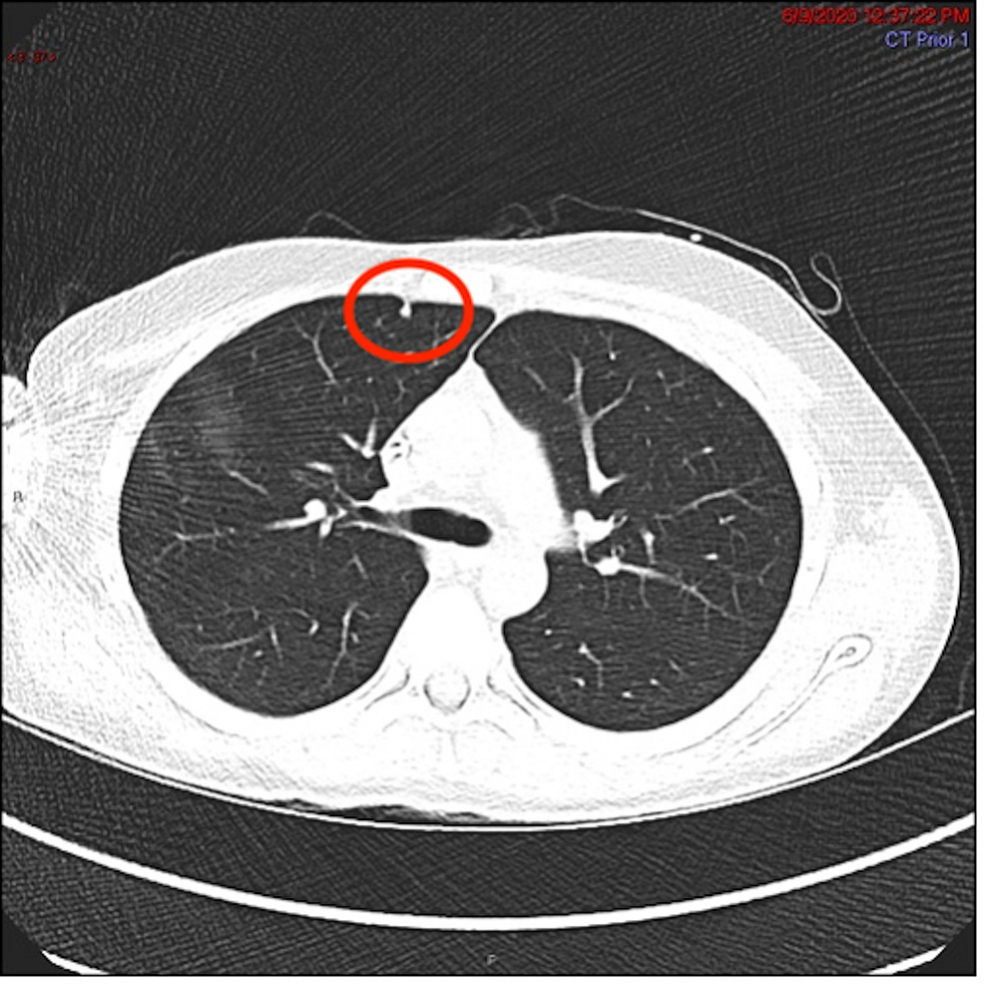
CT chest. Axial lung windows show a 5 mm pleural-based lung nodule in the anterior right upper lobe (red circle).
